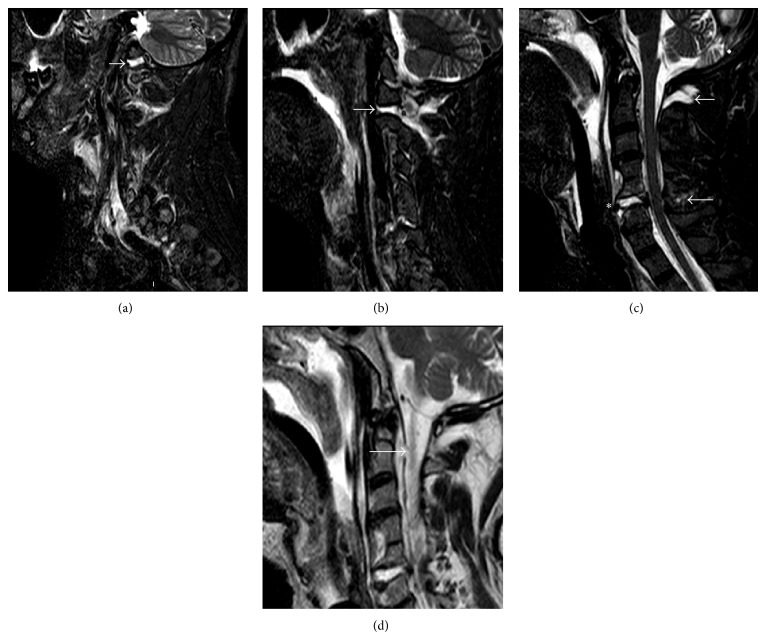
Multiple preoperative sagittal magnetic resonance images (MRI). (a) Left parasagittal short tau inversion recovery (STIR) image demonstrating abnormal fluid in the left atlantooccipital joint (arrow). (b) Left parasagittal STIR image demonstrating abnormal fluid in the left C1-C2 joint (arrow). (c) Midline sagittal STIR image demonstrating disk disruption at the C5-C6 level (∗) and abnormal signal demonstrating posterior ligamentous injury at the occiput-C1 level (rostral arrow) and at the C5-C6 level (caudal arrow). (d) Sagittal T2-weighted MRI demonstrating ventral dural defect at the level of C2 (arrow).
